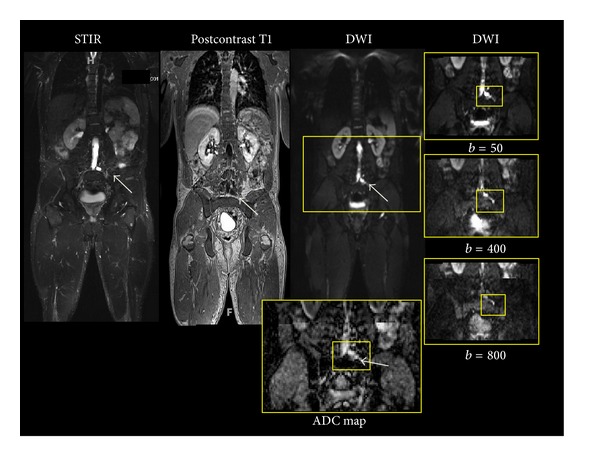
Whole body STIR, postcontrast T1-weighted, and DWI images with ADC map showing a paraspinal cyst (arrows) which is hyperintense on the STIR and DWI images, without demonstrable enhancement on the postcontrast image. The addition of contrast-enhanced sequences to a WB-MRI protocol enables the distinction of cysts from tumors in patients with neurofibromatosis, a distinction not otherwise possible by STIR and T1 imaging alone. In addition, the ADC value of the cyst was 2.6 × 10^−3^ mm^2^/sec in this patient, higher than the peripheral tumors identified in this patient (0.8 and 2.2 × 10^−3^ mm^2^/sec), suggesting that ADC values may provide an additional metric for the distinction of cysts and tumors.
